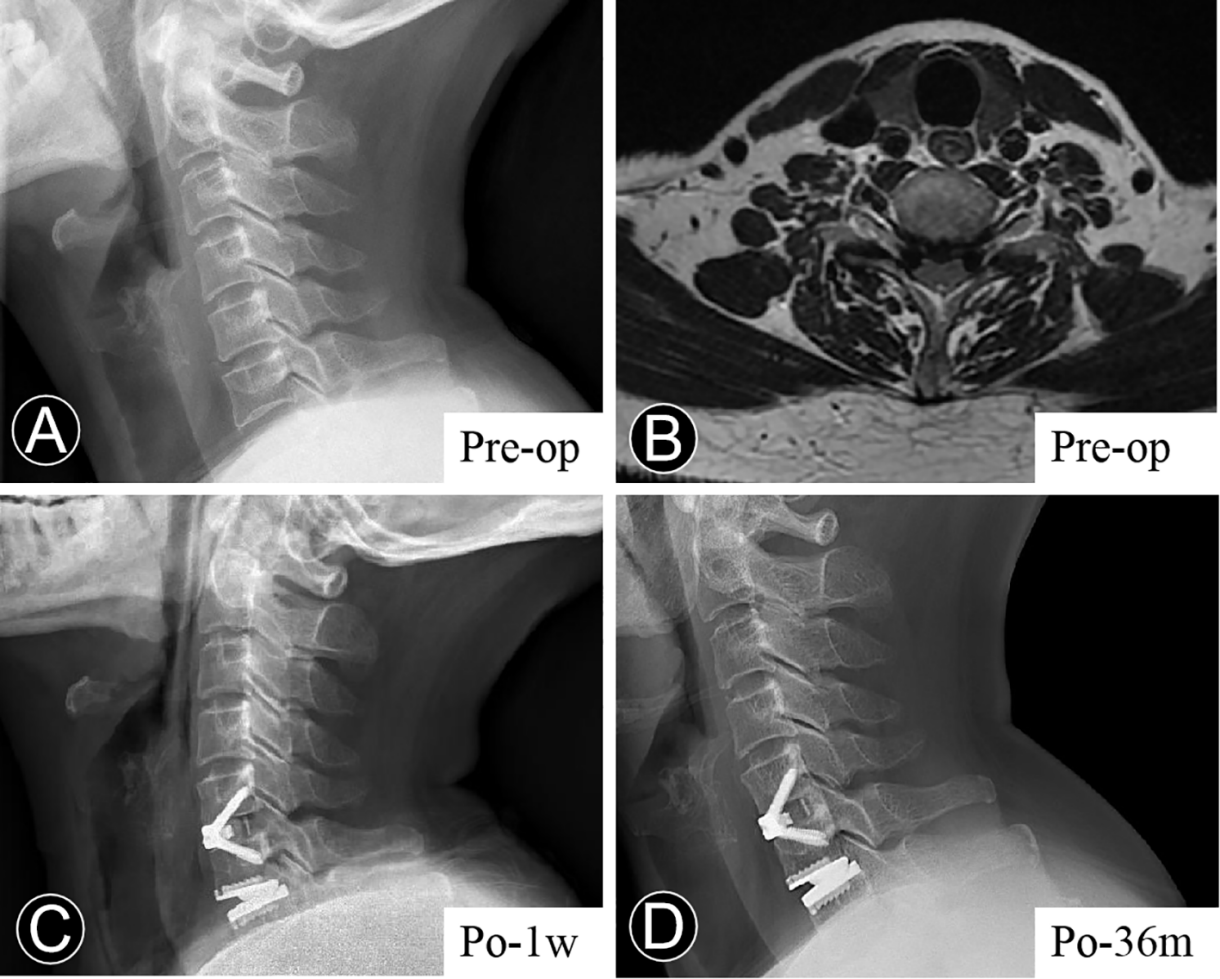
Representative case. Serial radiological examinations of a 67-year older woman with neck pain for more than two years. (A) Lateral view, showing that the preoperative cervical lordosis was 4.78°, and C2-7 sagittal vertical axis 4.01 cm. (B) T2-weighted axial MRI section, presenting a fatty infiltration degree of Goutalier 3-4. (C) The immediate postoperative lateral radiograph demonstrated a relatively satisfactory sagittal alignment. (D) At the last follow-up, the lateral radiograph demonstrated the loss of cervical lordosis and an increase in the sagittal vertical axis.
